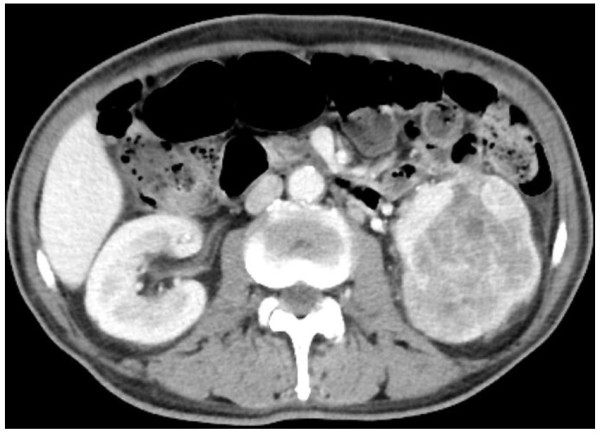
Abdominal enhanced computed tomography (CT) before axitinib treatment. (coronal section) Abdominal CT shows a hypervascular tumor (size, 9 × 8.5 cm) in the lower pole of the left kidney.
